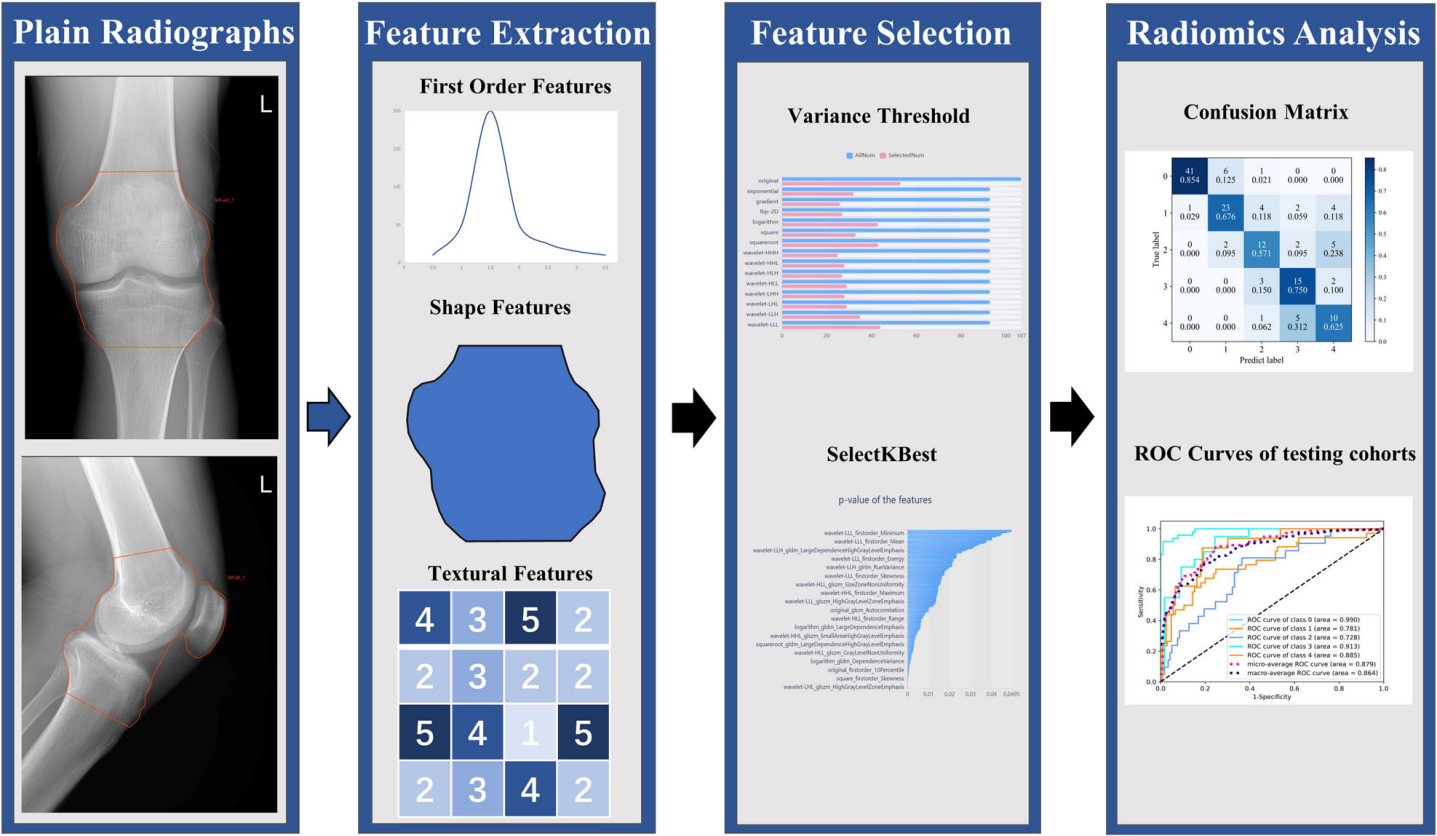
Regions of interest (ROIs) were manually delineated on AP&LAT plain radiographs. From these X-ray images, radiomic features were then extracted by quantifying their intensity, shape, and texture. The feature selection process was conducted in two stages. Subsequently, logistic regression (LR) models were employed to assess the grading performance of the selected radiomic features. The accuracy of the model was evaluated using receiver operating characteristic (ROC) curves
